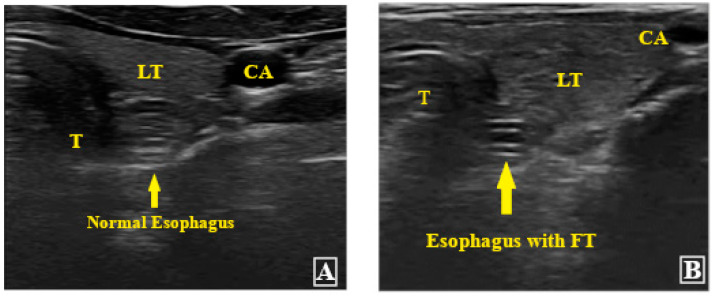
Neck point ultrasonography image (CA = carotid artery, LT = left thyroid lobe, T = trachea). The normal esophagus without the feeding tube (FT) (A) vs. with the FT (B).
